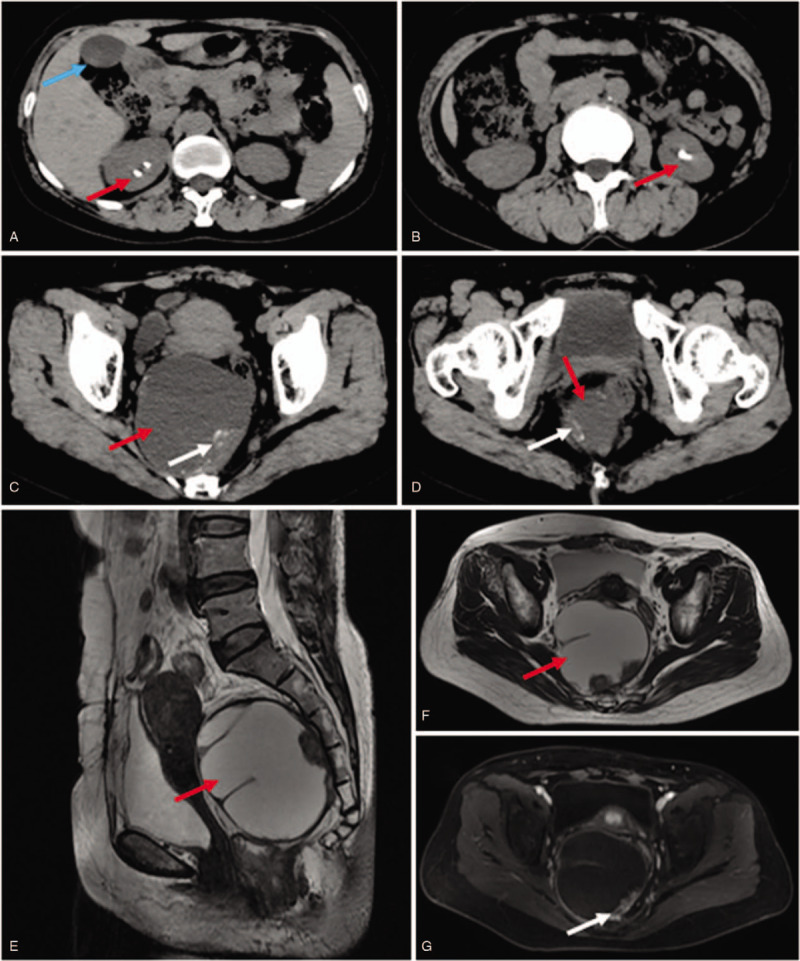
Imaging examination. (A) CT showing gallstones (blue arrow) and right kidney stones (red arrow). (B) The left kidney stone (red arrow). (C) CT showing a 80 × 80 mm sized mass with low density shadows in the pelvic cavity (red arrow). The white arrow indicates the high-density signals within the lesion. (D) The uterus and rectum were compressed. (E) MRI showing a large cystic shadow (red arrow) with long T1 and T2 signals. (F) The boundary between the lesion and the right accessory was unclear. A red arrow indicates the lesion. (G) The separation wall and the nodules (white arrow) of the lesion were significantly enhanced. CI = computed tomography, MRI = magnetic resonance imaging.
